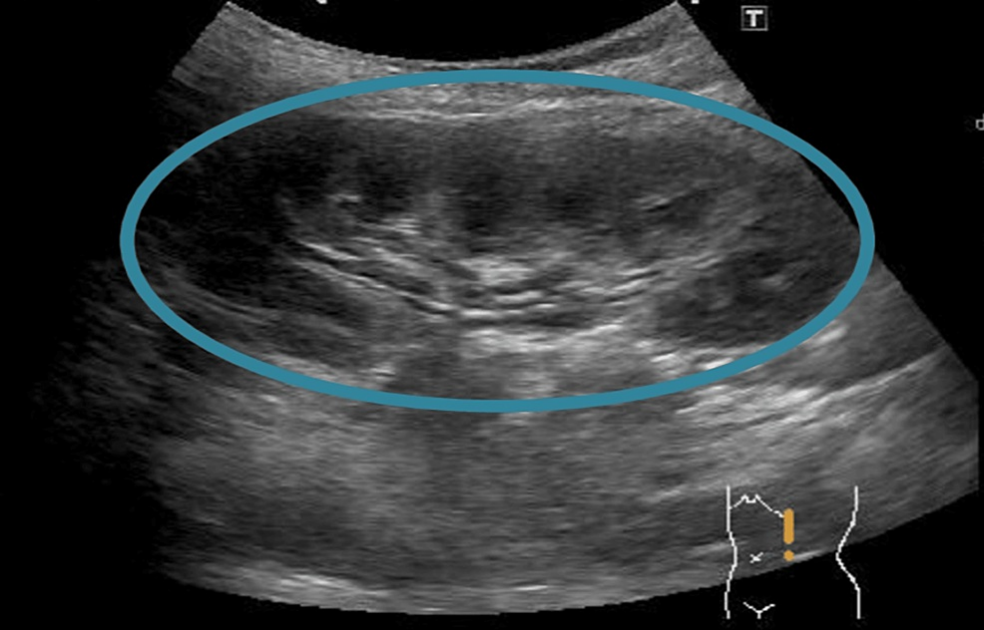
Abdominal POCUS finding (left abdominal short axis view) Ultrasonography showed a kidney with mild hydronephrosis (circle), but free peritoneal fluid was not detected. POCUS: Point-of-care ultrasound.
